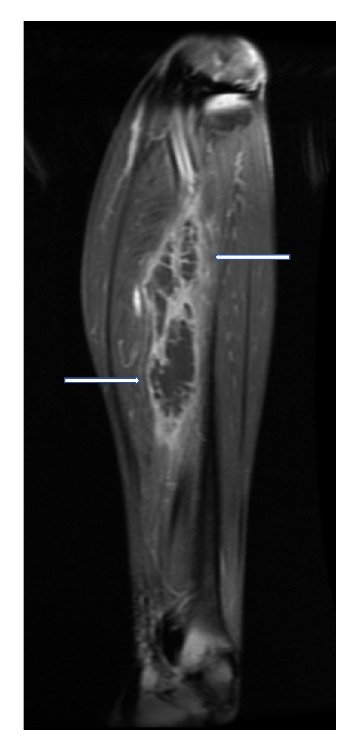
T2 weighted, fat saturated, postcontrast, coronal MR image of left calf showing a multiloculated fluid collection with associated enhancing rim and septations (white arrows) within the tibialis posterior muscle, compatible with an intramuscular abscess.
